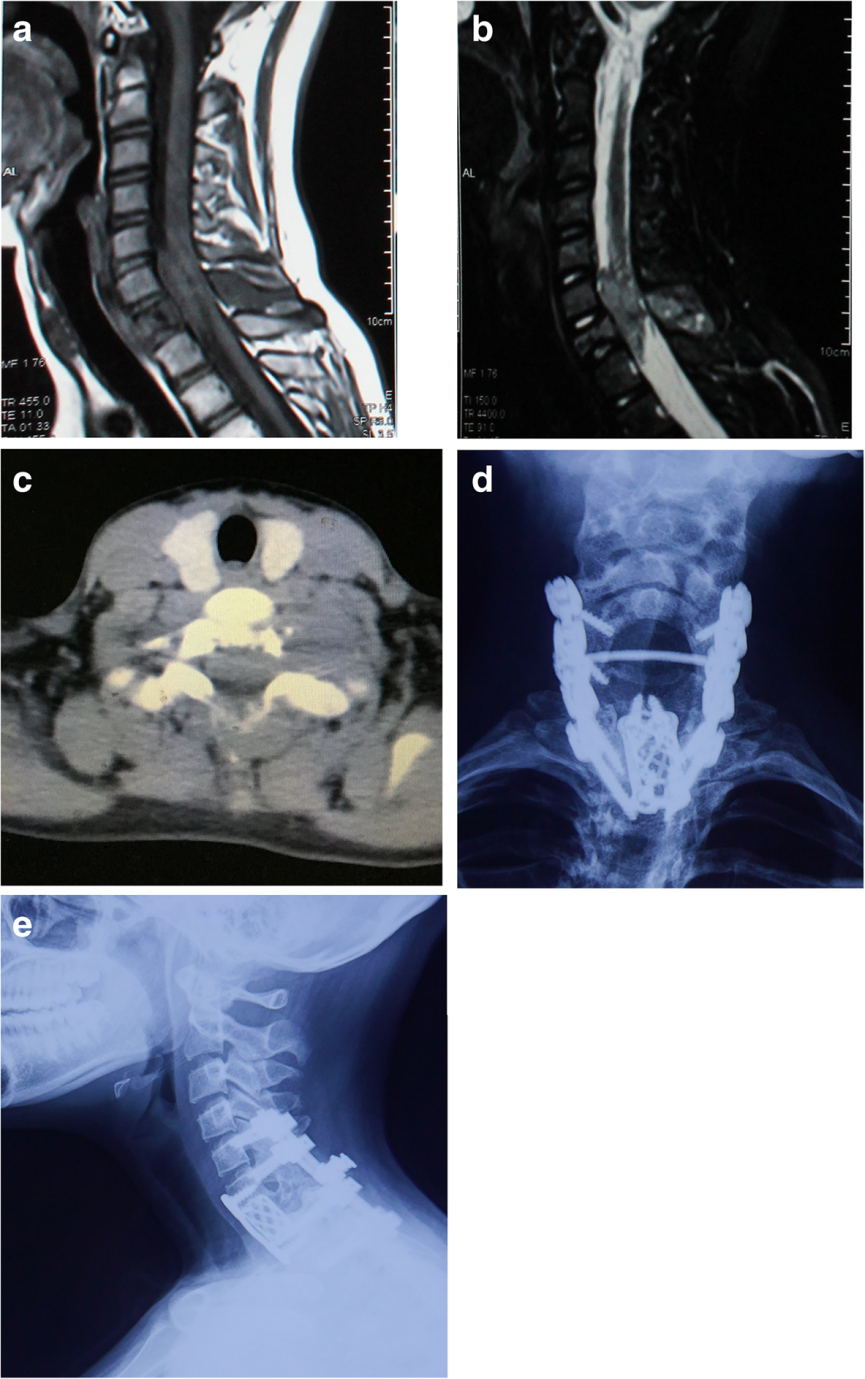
Patient no. 13: MRI showing low signals in T1- (a) and heterogeneous signals in T2 (b)-weighted images of a soft tissue mass in the C7 vertebral body and appendix, with spinal cord compression from C6 to T1. CT images (c) showing a destructive soft tissue mass and osteolytic lesion in the C7 vertebral body and appendix. Cervical vertebra anterior and posterior (AP) (d) and lateral (LAT) (e) X-rays at the follow-up 52 months after surgery, demonstrating the maintenance of the instruments and spinal stability
